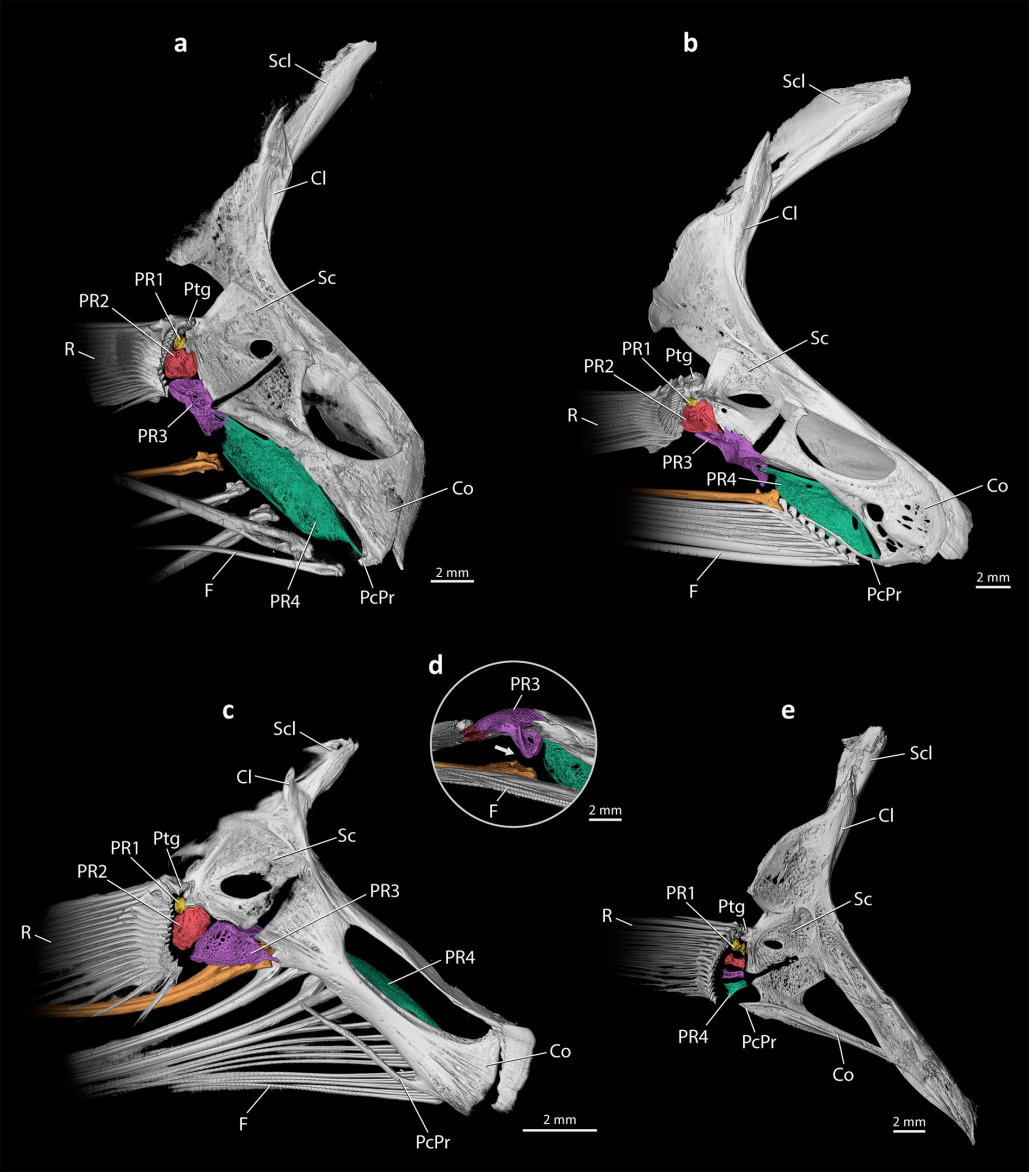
X-ray microcomputed tomographies of left pectoral girdle in medial view; (a) *Pentanemus quinquarius*, Polynemidae; (b) *Polydactylus opercularis*, Polynemidae; (c) *Polynemus multifilis*, Polynemidae; (d) ventral view of pectoral radial 3 of *P. multifilis*, Polynemidae; (e) *Cynoscion striatus*, Sciaenidae. Cl, cleithrum; Co, coracoid; F, pectoral filaments; PcPr; postcoracoid process; PR1–4, pectoral radial 1–4; Ptg, propterygium; R, pectoral-fin rays; Sc, scapula; Scl, supracleithrum. Arrow indicates site of articulation between pectoral radial 3 and the dorsalmost pectoral filament in (d); postcoracoid process broken distally in (a).
